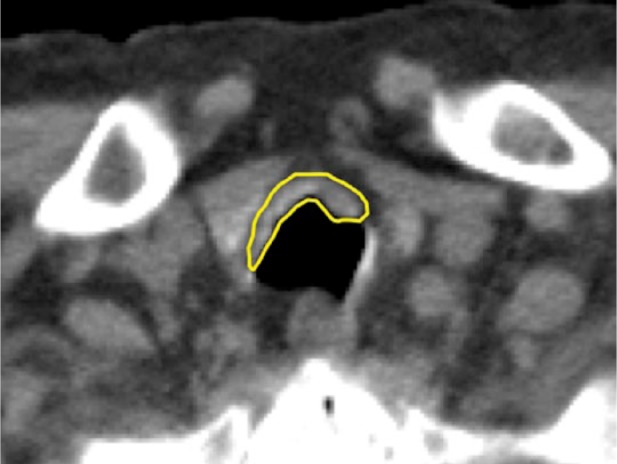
The chest CT shows a subglottic mass and anterior tracheal wall thickening with some calcifications, circumscribed by the yellow line.
